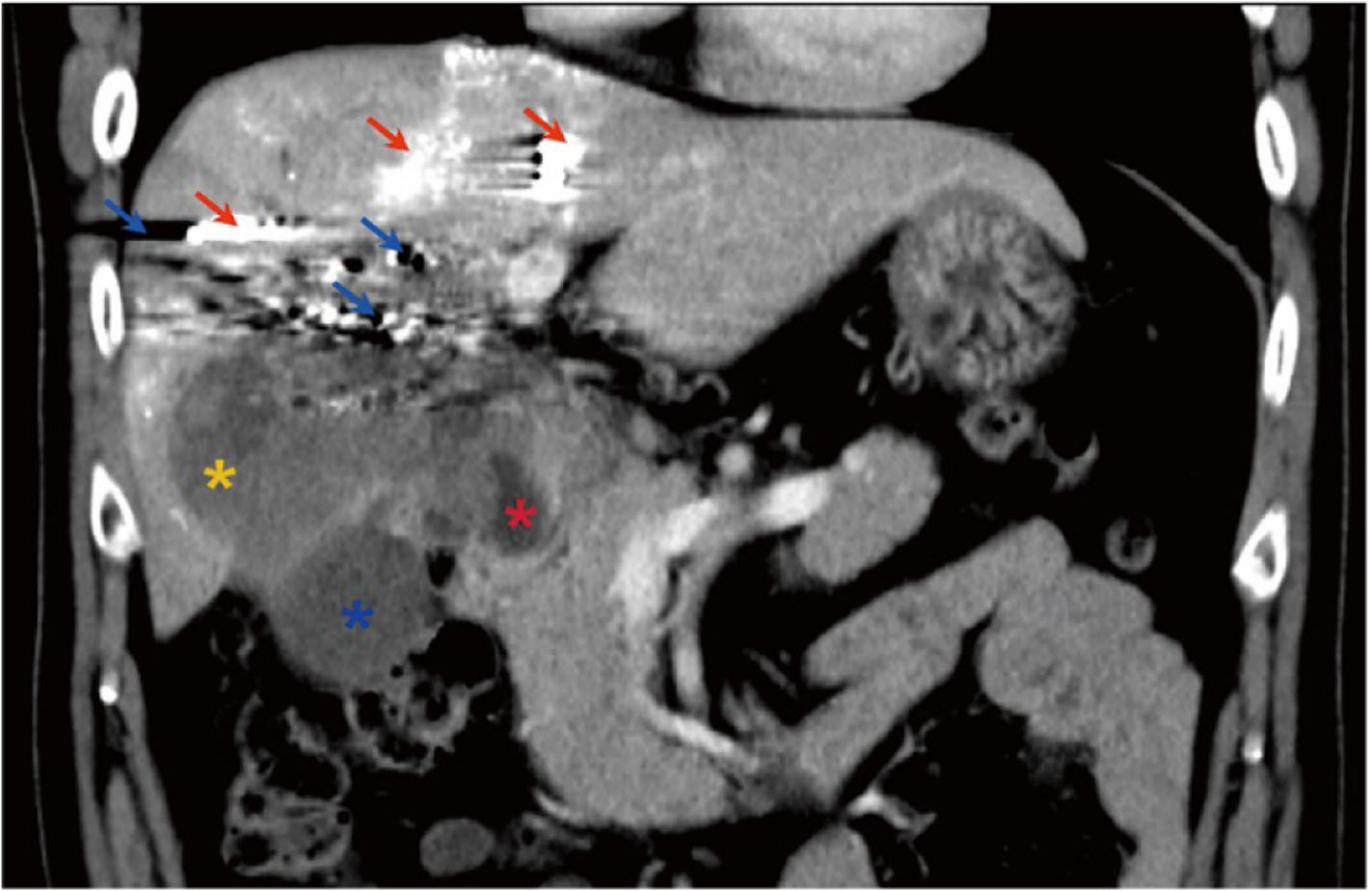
CT scan showing the mass located in the gallbladder, liver, and duodenum. The blue asterisk represents the non-tumor fundus of the gallbladder. The yellow asterisk represents the liver parenchyma infiltrated by the tumor. The red asterisk represents the tumor protruding into the duodenal lumen. The red arrows represents iodipin on CT images. The blue arrows represents CT artifacts
